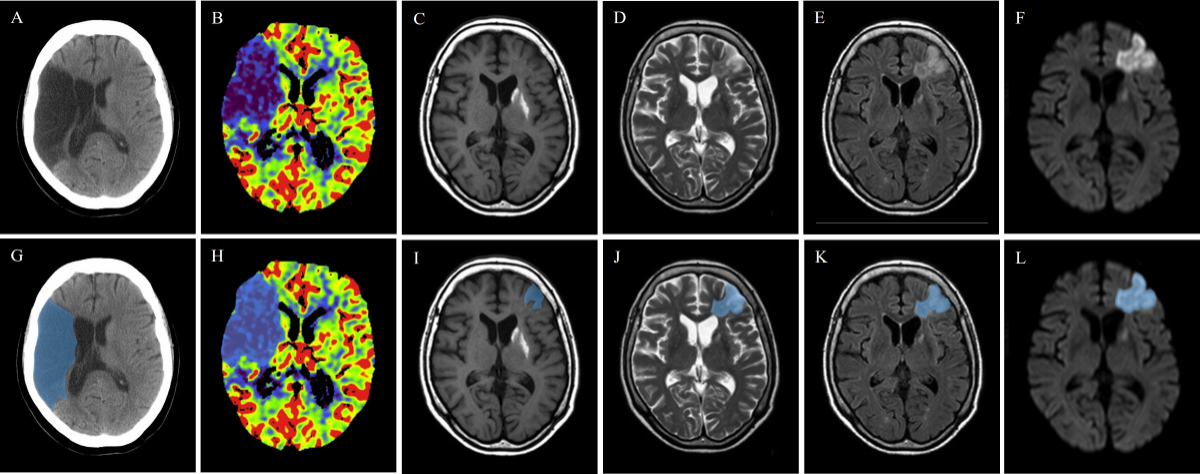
Stroke lesions identified using different imaging modalities. (A) Noncontrast computed tomography (NCCT). (B) CT perfusion (CTP). (C) T1-weighted imaging (T1WI). (D) T2-weighted imaging (T2WI). (E) Fluid-attenuated inversion recovery (FLAIR). (F) Diffusion-weighted imaging (DWI). (G) NCCT (annotated). (H) CTP (annotated). (I) T1WI (annotated). (J) T2WI (annotated). (K) FLAIR (annotated). (L) DWI (annotated). Stroke lesion areas are marked in blue, and the images are not paired.
